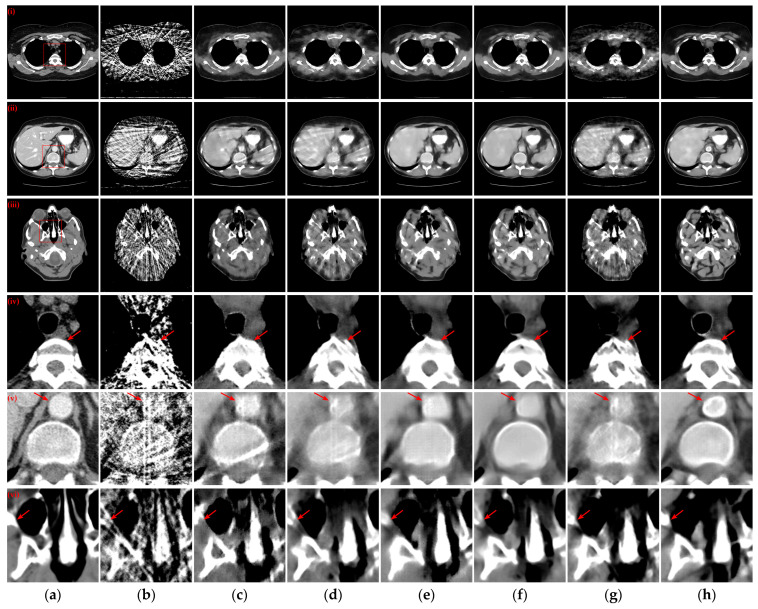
The SVCT reconstruction results from different methods with 32 projection views. (a) The reference image; (b) FBP; (c) MWNet; (d) DuDoTrans; (e) DDPTransformer; (f) MIST; (g) RegFormer; (h) Ours. (i)–(iii) are the chest CT, abdominal CT, and head CT, respectively, and (iv)–(vi) correspond to the enlarged ROIs marked by the red boxes in the reference images above. The display window is [−160, 240] HU.
